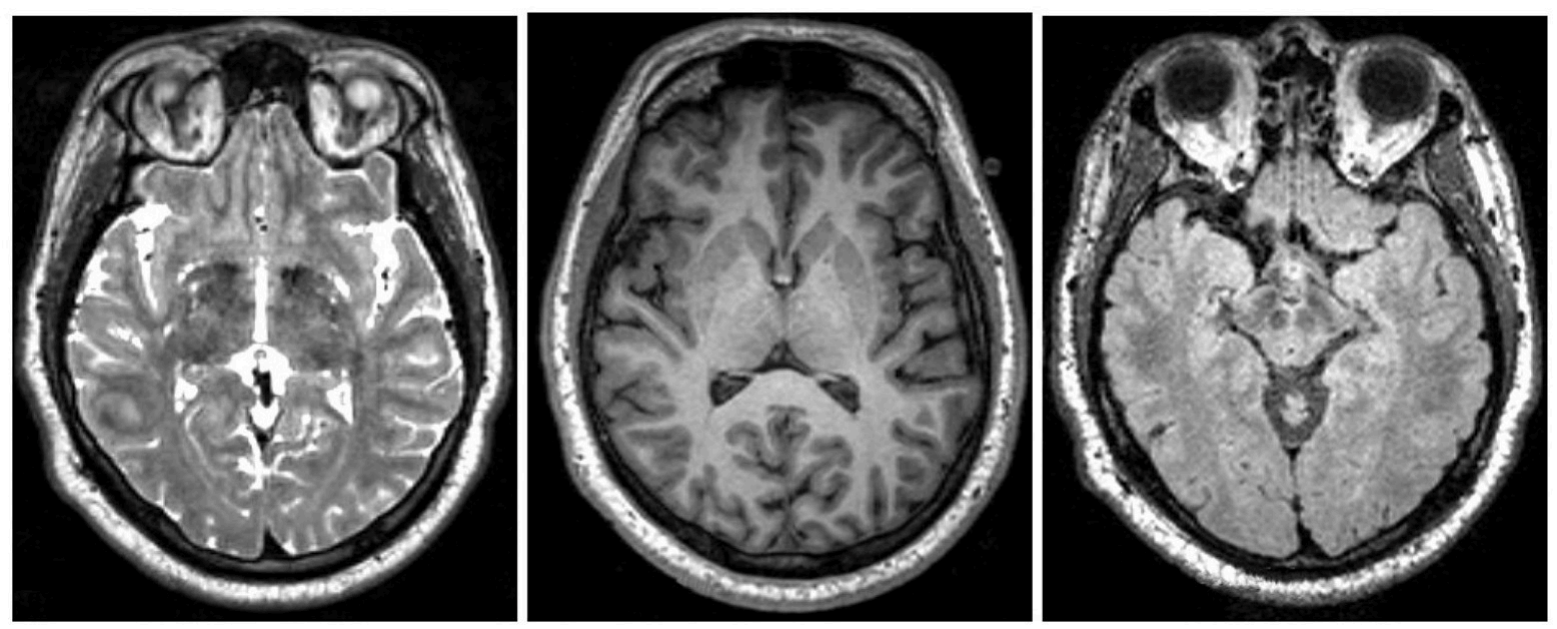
Examples of different MRI imaging techniques. Left: T2 image. Middle: T1 image. Right: FLAIR image. Table shows the brightness of the tissue using the three most common MRI imaging technique from dark to bright (Preston, 2006).
